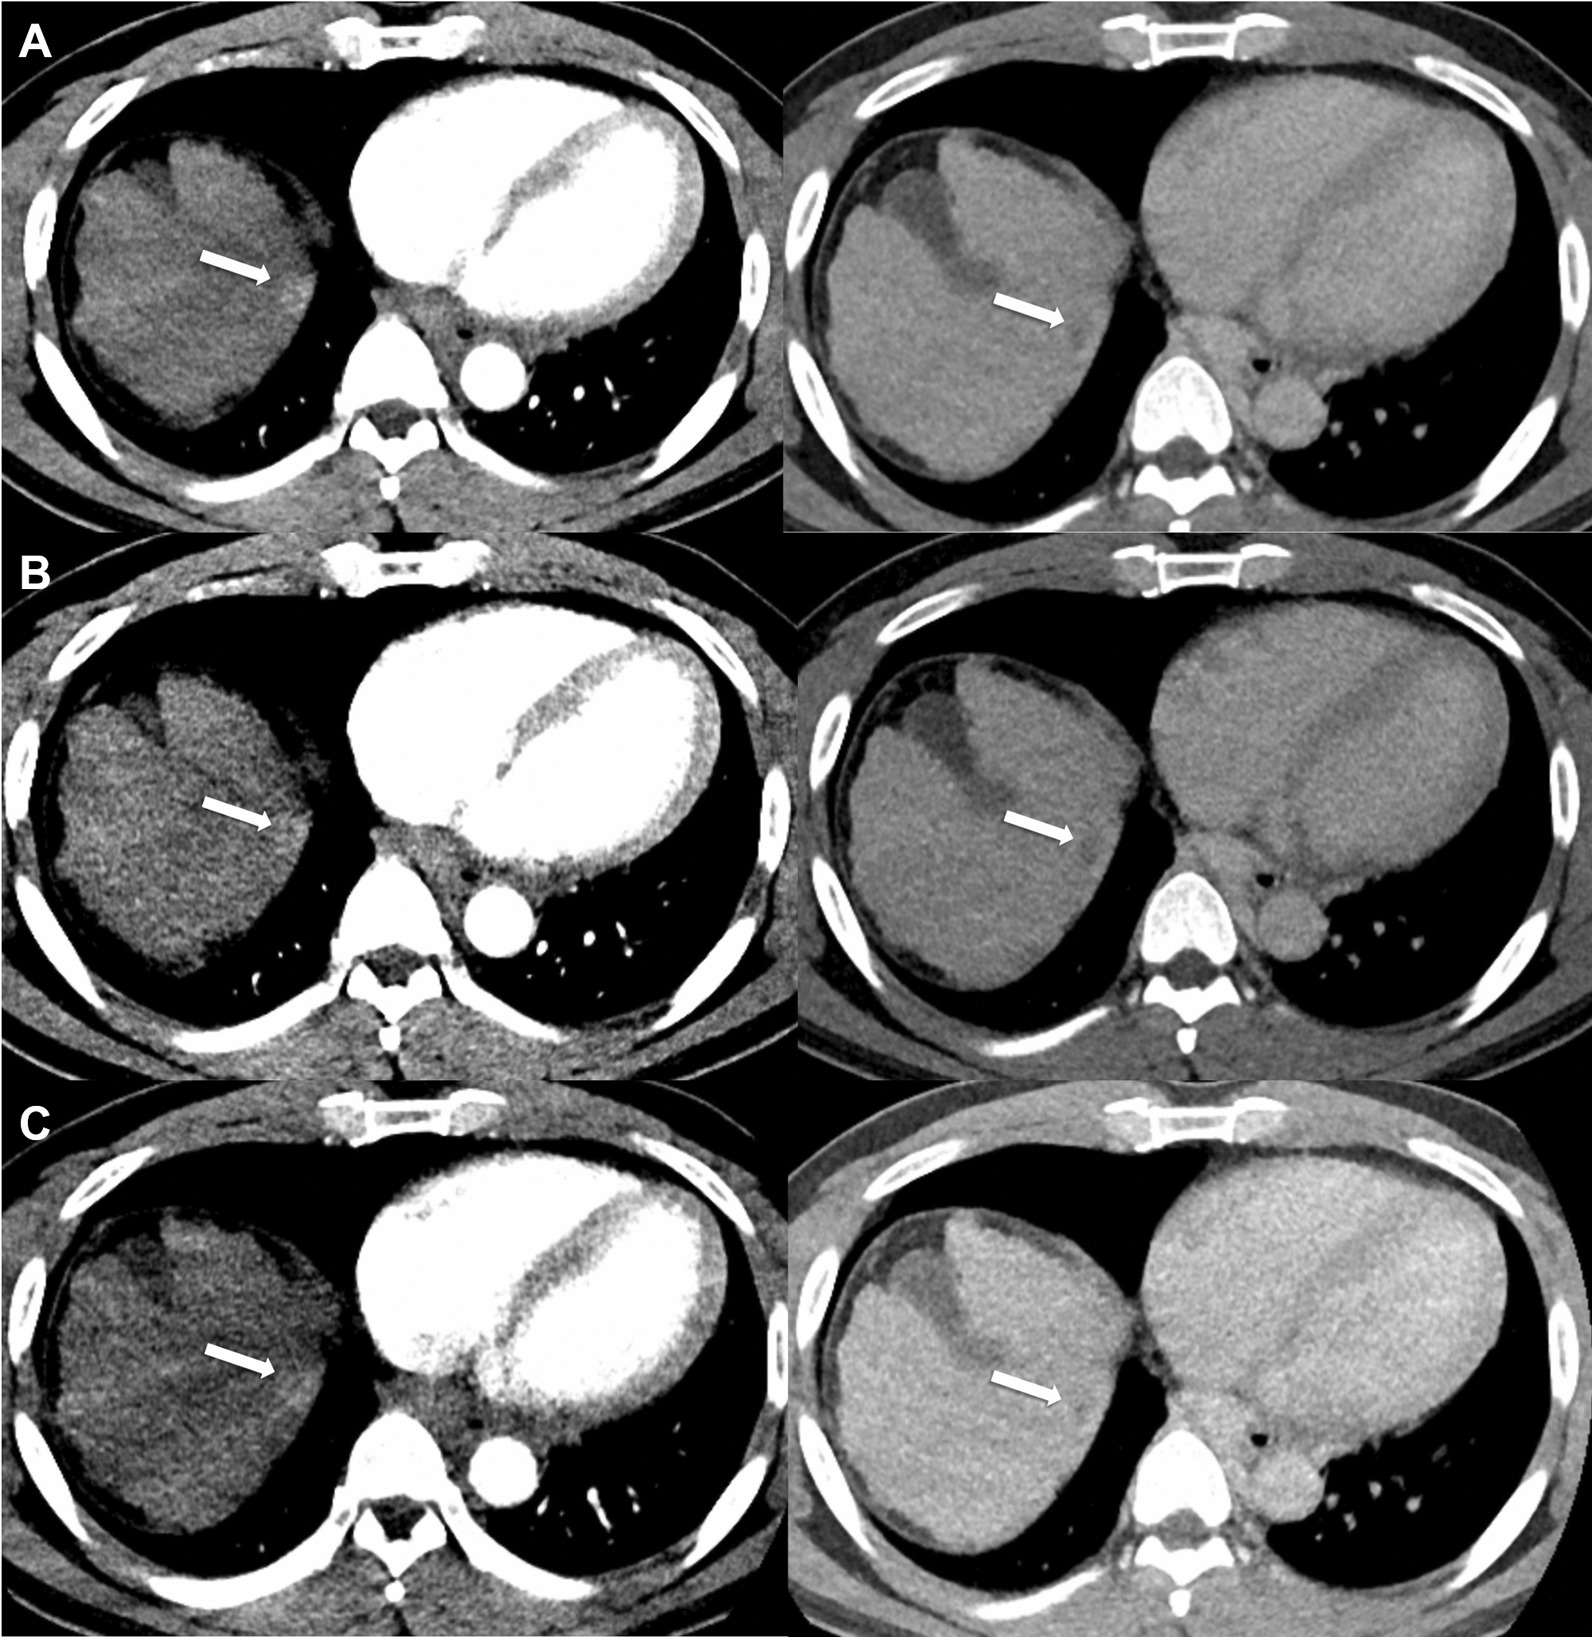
A 1.5-cm sized hepatocellular carcinoma in a 45-year-old man with 28.2 kg/m^2^ body mass index. A Standard dose CT (17.1 mSv) arterial and delayed phase, B low-dose CT (12.0 mSv) arterial and delayed phase, and C ultra-low-dose CT (5.1 mSv) arterial and delayed phase. On SDCT (A), both readers detected the lesion as hepatocellular carcinoma. However, reviewer 1 could not detect the lesion on LDCT (B) and ULDCT (C). Reviewer 2 detected the lesion but was characterized as benign (LI-RADS 3)on LDCT (B) and ULDCT (C). Reviewer 1 and reviewer 2 rated the overall image quality as 5 and 4 respectively for SDCT (A), 3 and 4 respectively for LDCT (B), and 2 and 4 respectively for ULDCT(C)
